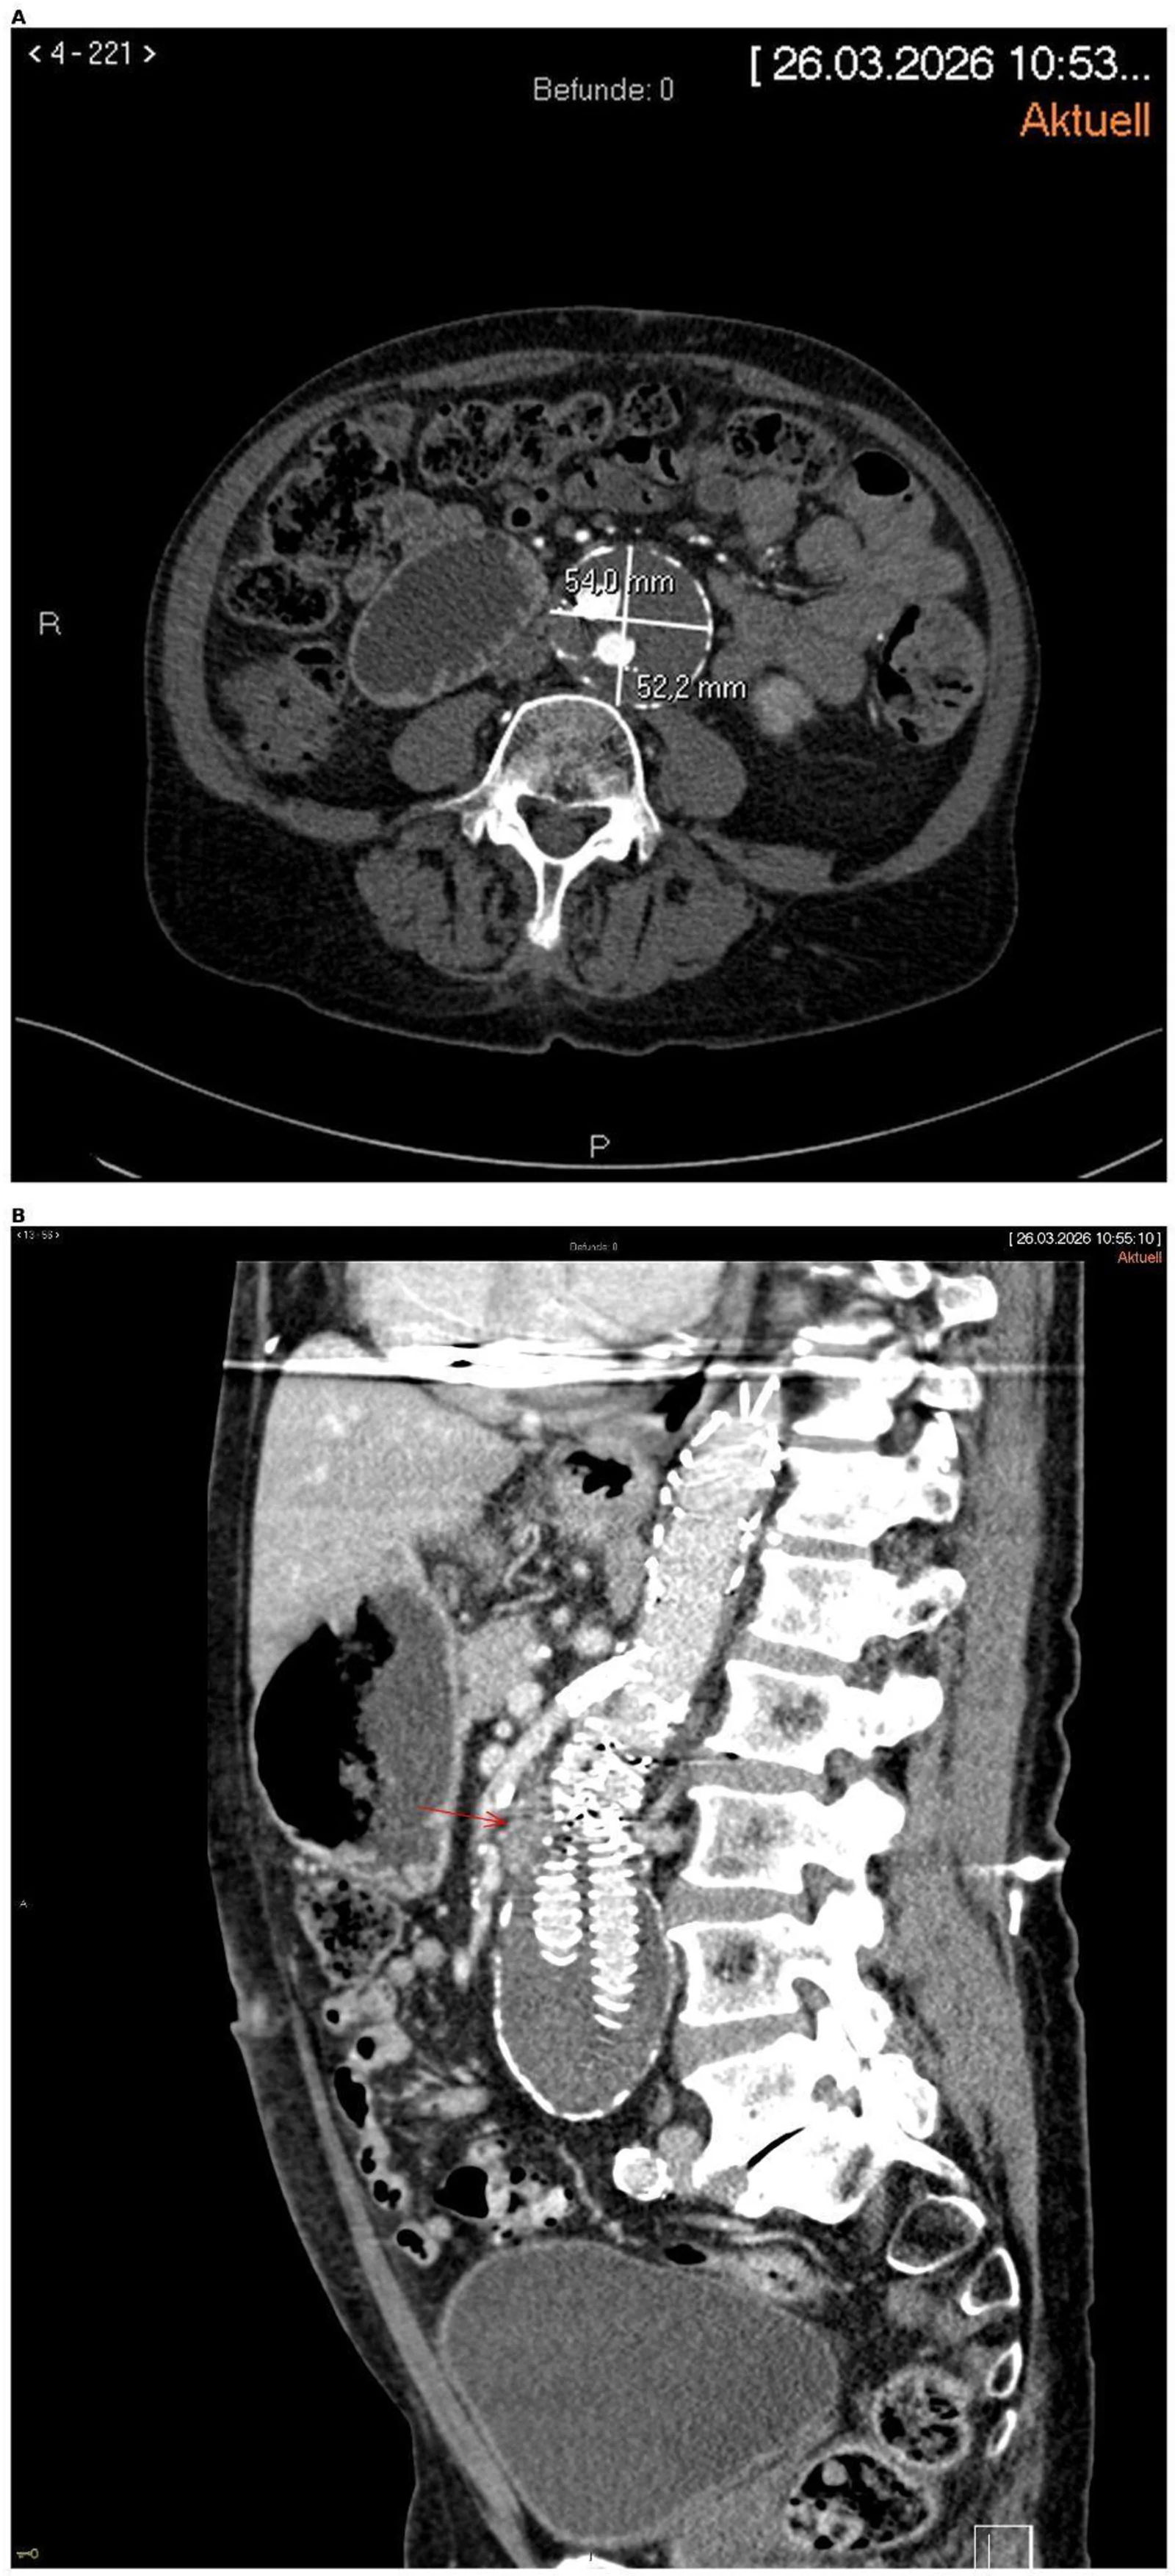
Axial (A) and sagittal (B) contrast-enhanced CT reconstructions from 2026 demonstrating the anatomical relationship between the infrarenal abdominal aortic aneurysm and the third portion of the duodenum. The aneurysm measures approximately 51 × 52 mm (A). The sagittal reconstruction (B) demonstrates focal compression of the third portion of the duodenum at the level of the aneurysmal sac (red arrow).
